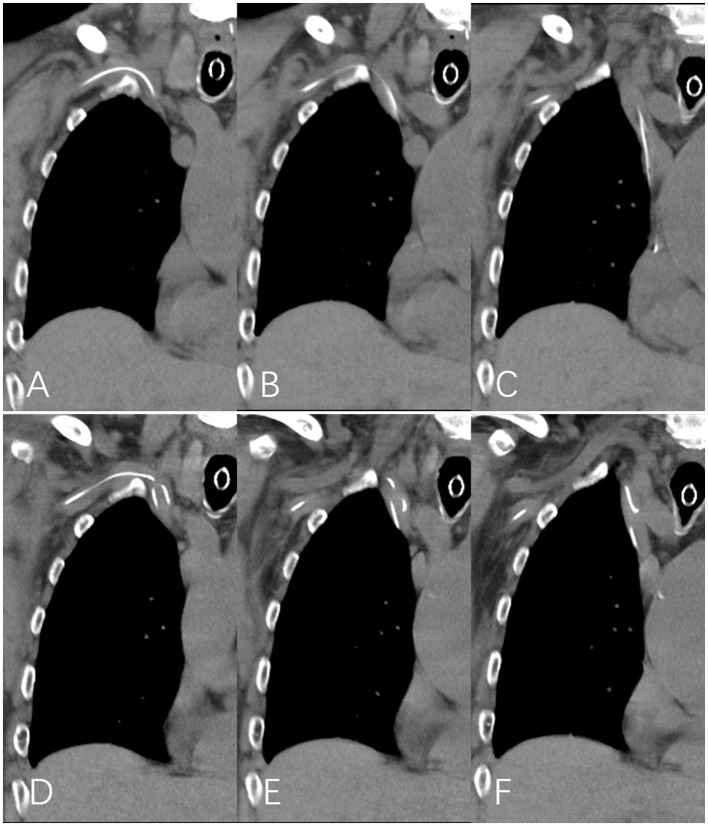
The catheter appearances on chest CT scans. (A–C) The PICC line tip position and shape were confirmed to be normal on March 28. (D–F) The proximal PICC segment formed a reverse S-shaped kink in the right brachiocephalic vein on April 2, with the tip displaced to the upper third of the superior vena cava.
